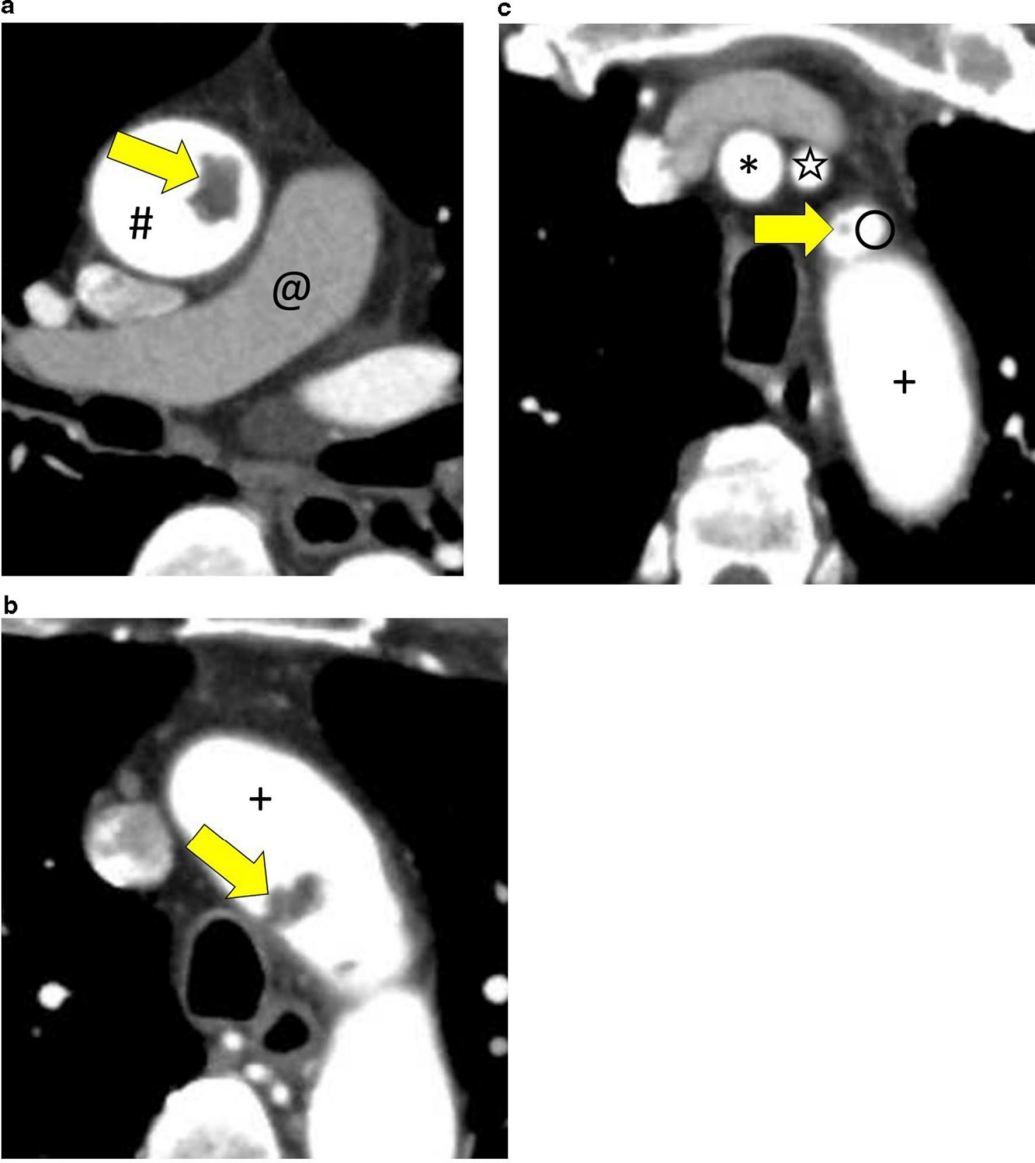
CT images. a An axial CT image showing the thrombus in the ascending aorta (arrow). b An axial CT image showing the thrombus in the aortic arch (arrow). c An axial CT image showing the thrombus in the left subclavian artery (arrow). # Ascending aorta, @ pulmonary artery, + aortic arch, * brachiocephalic artery, open star left common carotid artery, open circle left subclavian artery
